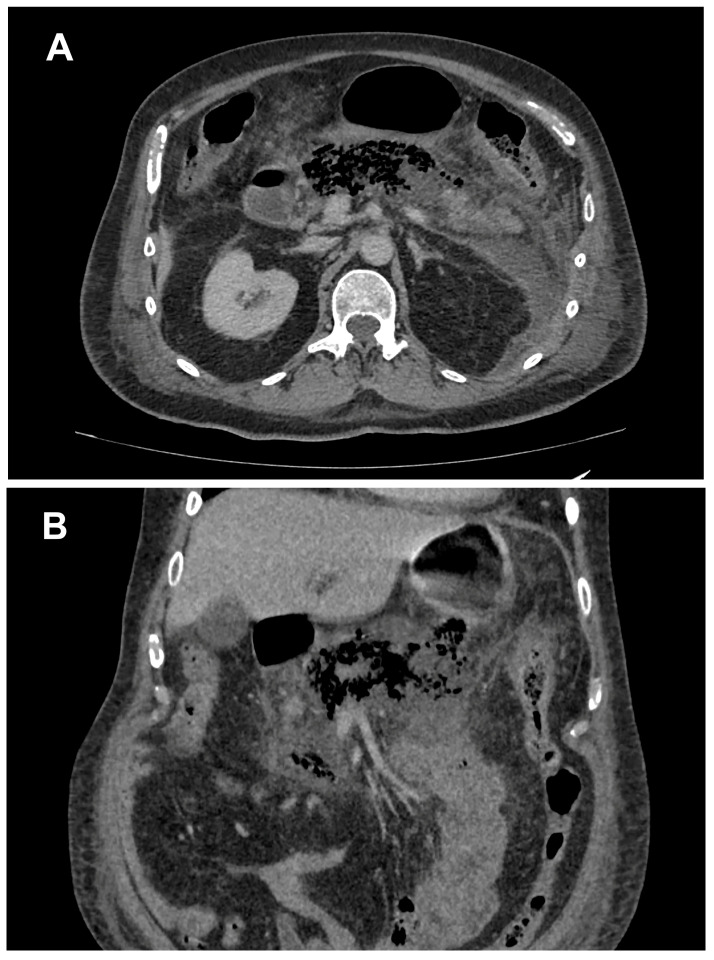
Contrast-enhanced computed tomography, axial (A) and coronal (B) reconstruction, venous phase of the exam showing gas in the body and tail of the pancreas. Pancreatic exudate in the left anterior pararenal space with surrounding retroperitoneal fat stranding is also noted.
